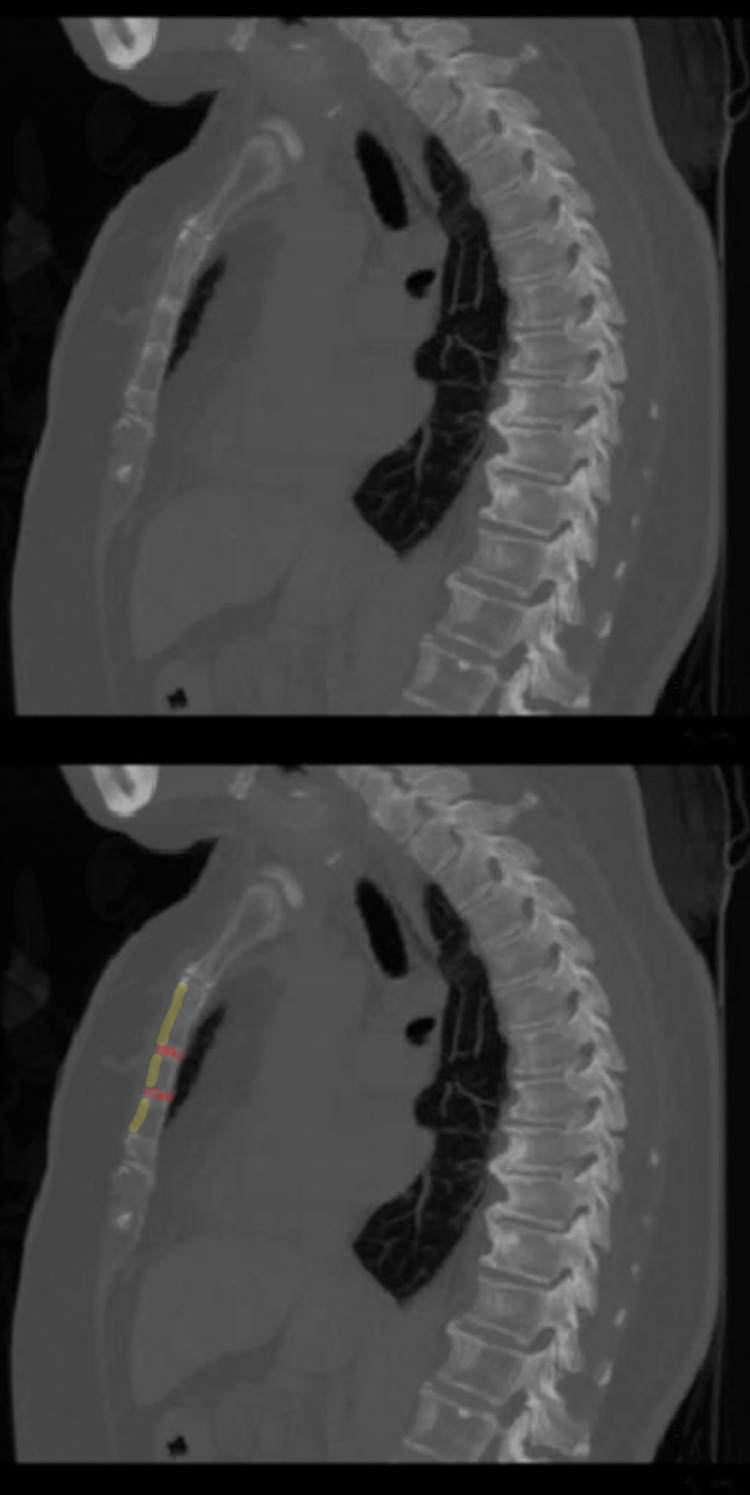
Sagittal view of the chest computed tomography. The anterior cortex of the sternum (yellow) and the fracture sites (red) are highlighted.
